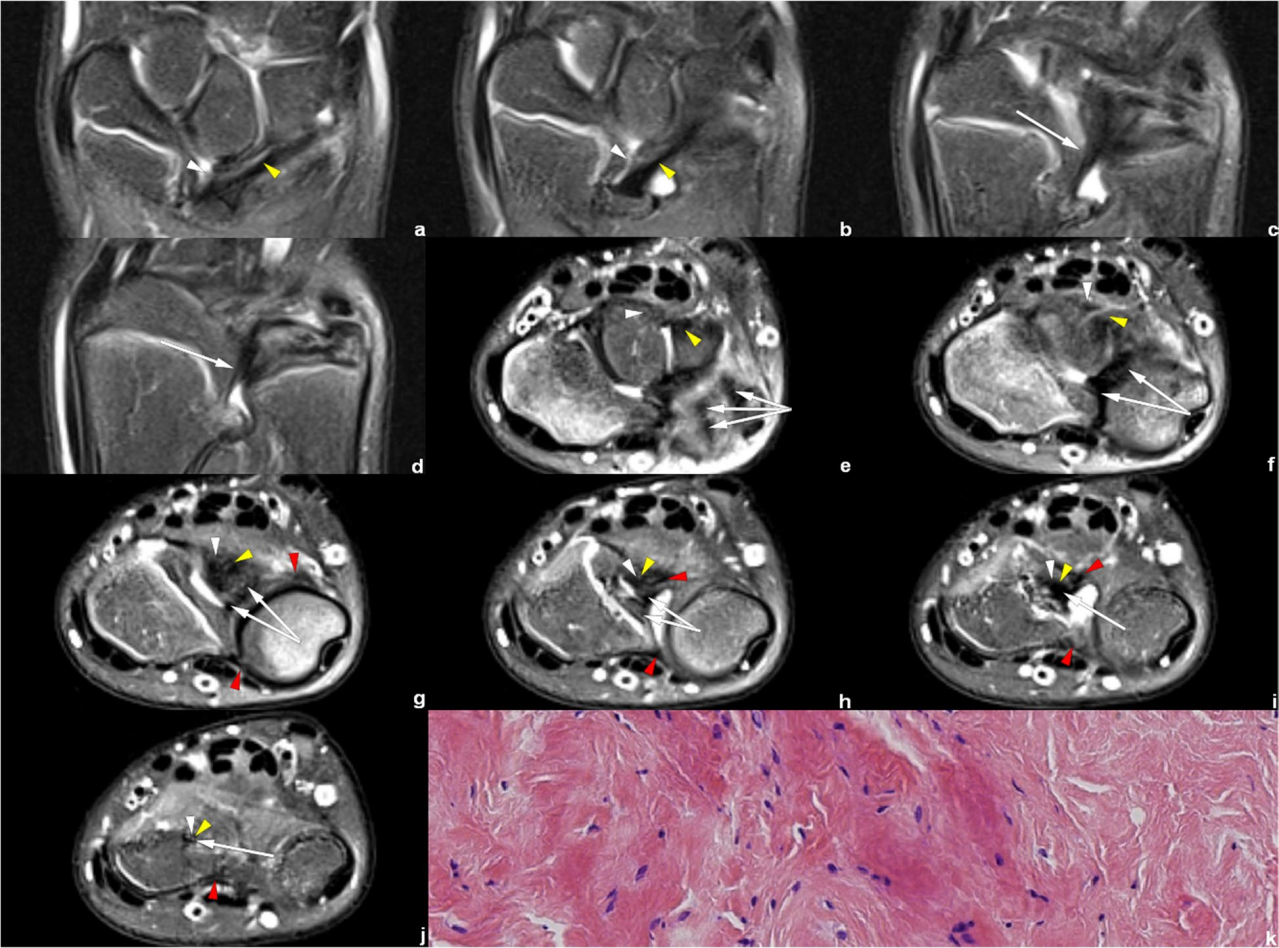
Left wrist MRI of the patient No. 0919. a–d Coronal PDWI-FS images show the VL (white arrowhead), RTL (yellow arrowhead), and CD (white arrow). e–j Axial PDWI-FS images show the CD (white arrow), volar and distal radioulnar ligament (red arrowhead), VL (white arrowhead), and RTL (yellow arrowhead). k The postoperative pathological findings show dense fibrous connective tissue
